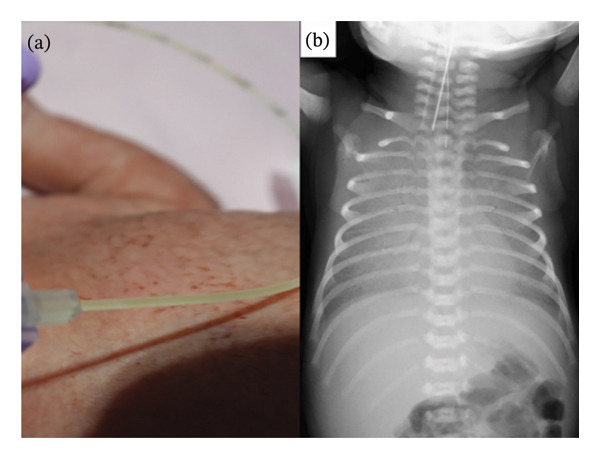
(a) Small, well‐circumscribed red spots on the patient’s trunk. (b) Chest radiograph of the patient on admission to the neonatal intensive care unit. Widespread opacification throughout both lung fields is seen even after lung surfactant had been administrated.
